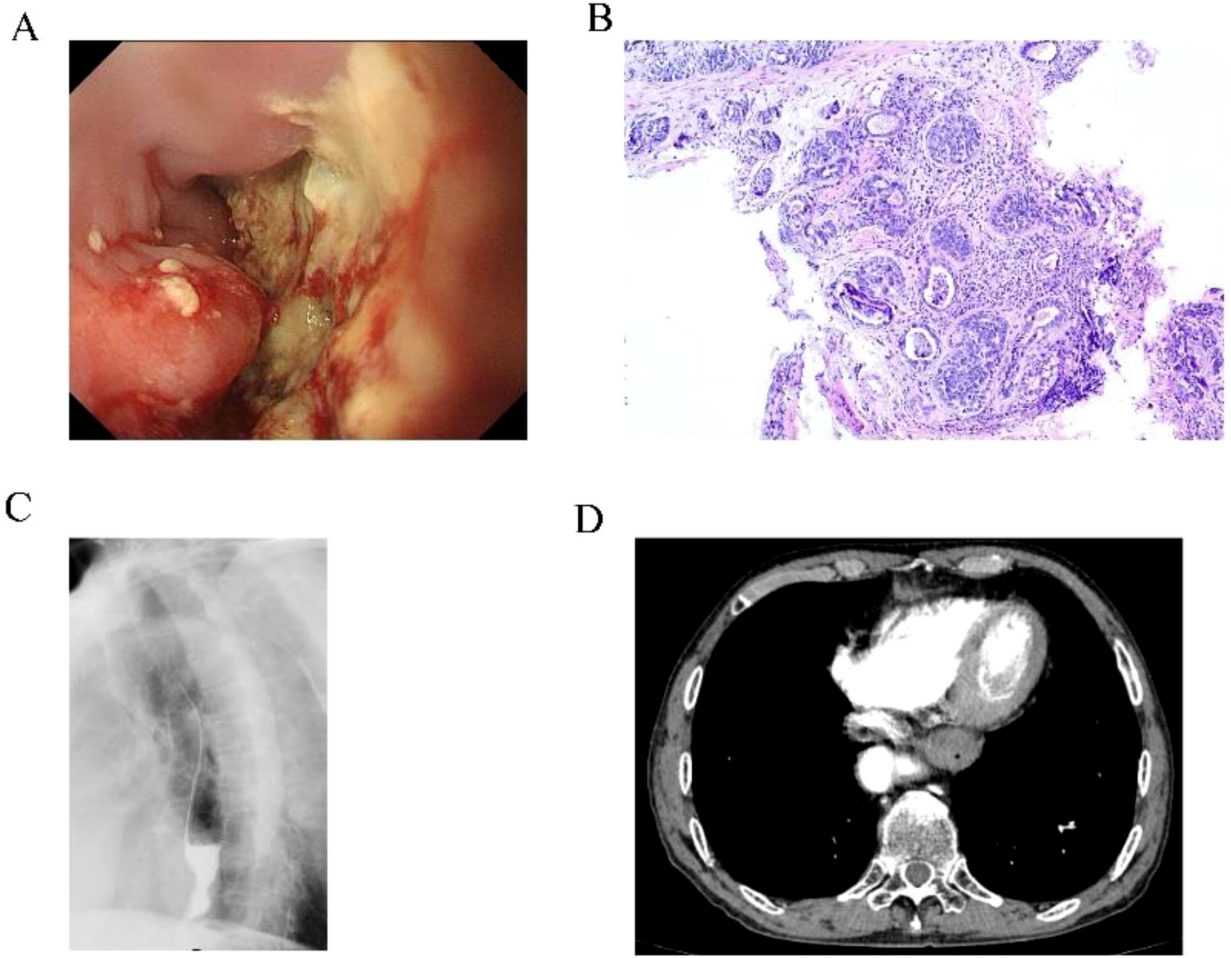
Pre-treatment examination results of the patients. (A) Gastroscopy results showed neoplasm in the lower esophagus. (B) The lesion site of the esophagus showed squamous cell carcinoma. (C) Gastrointestinal angiography showed stenosis of the lower esophagus. (D) Chest CT showed thickening of the lower wall of the esophageal tube with stenosis.
